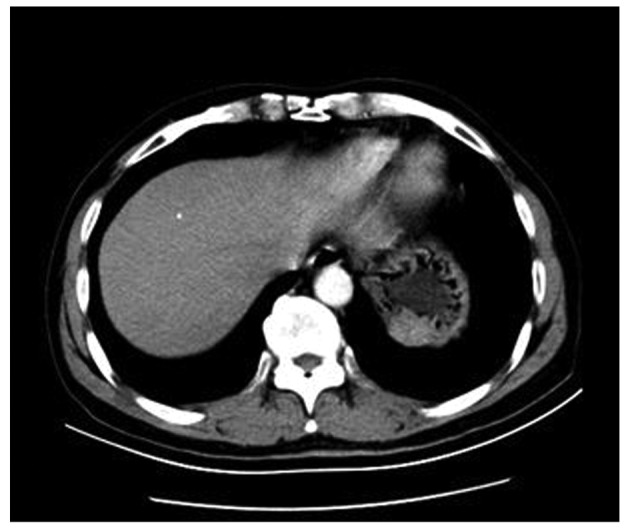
Abdominal contrast-enhanced computed tomography showed a well-marginated ovoid mass of ~2.6×1.9 cm in size located close to the gastric fundus.
